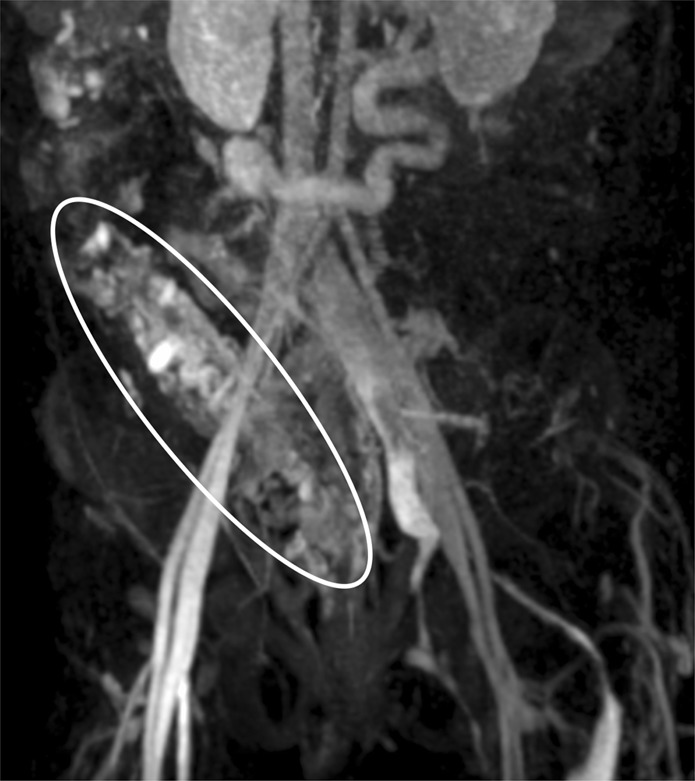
TWIST MRI of a 10-year old girl with expanded sigmoidal VM. Circle: Massively dilated vessels with venous flow characteristics.
